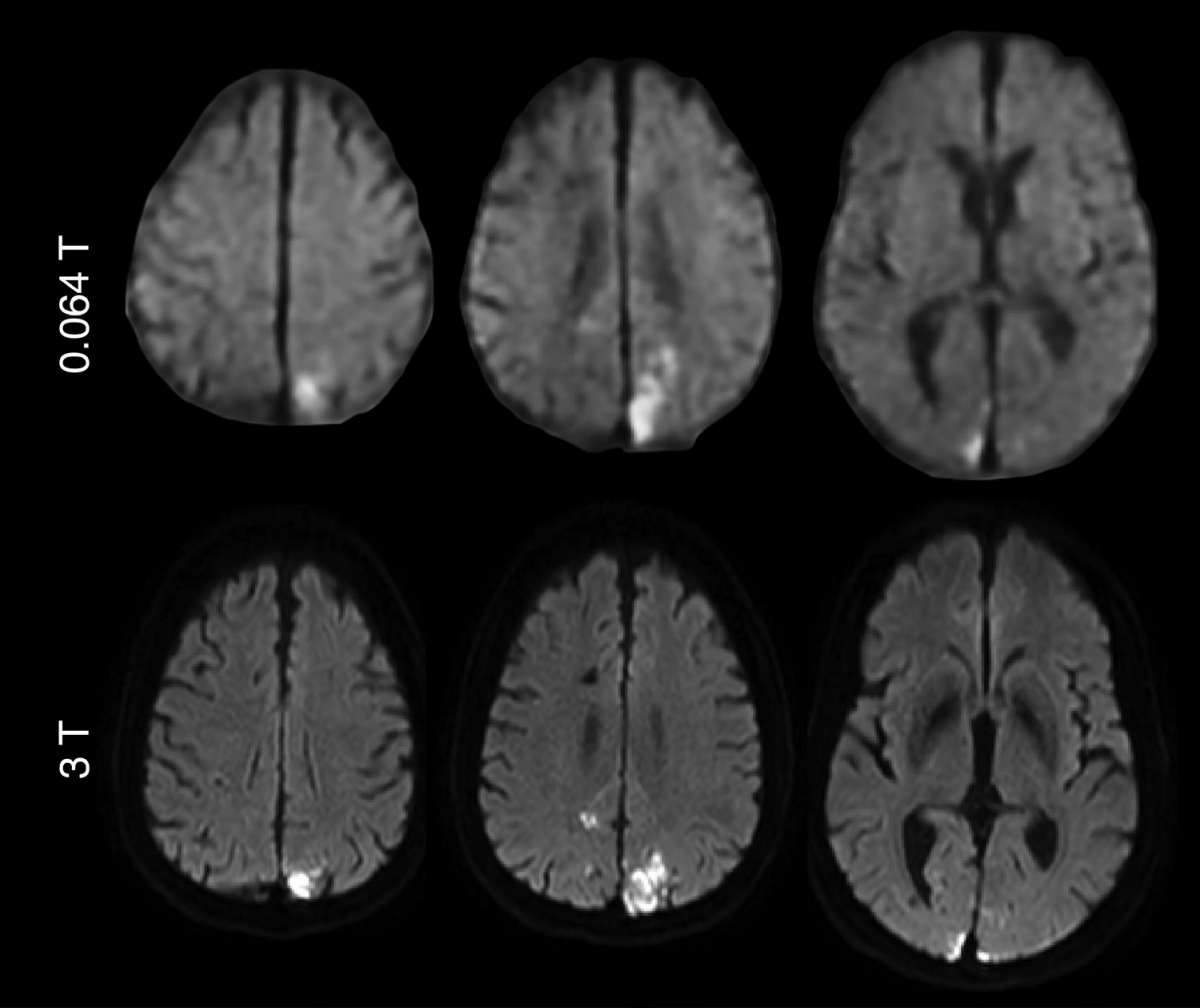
Averaged trace diffusion-weighted imaging (DWI) on the next-generation v2 system. Comparison between low-field trace DWI on the v2 system against conventional 3 T counterparts in a patient with bilateral acute ischemic infarcts in the occipital lobe. The skull has been stripped for visualization purposes. Each image shown at 0.064 T is from v2 hardware.
